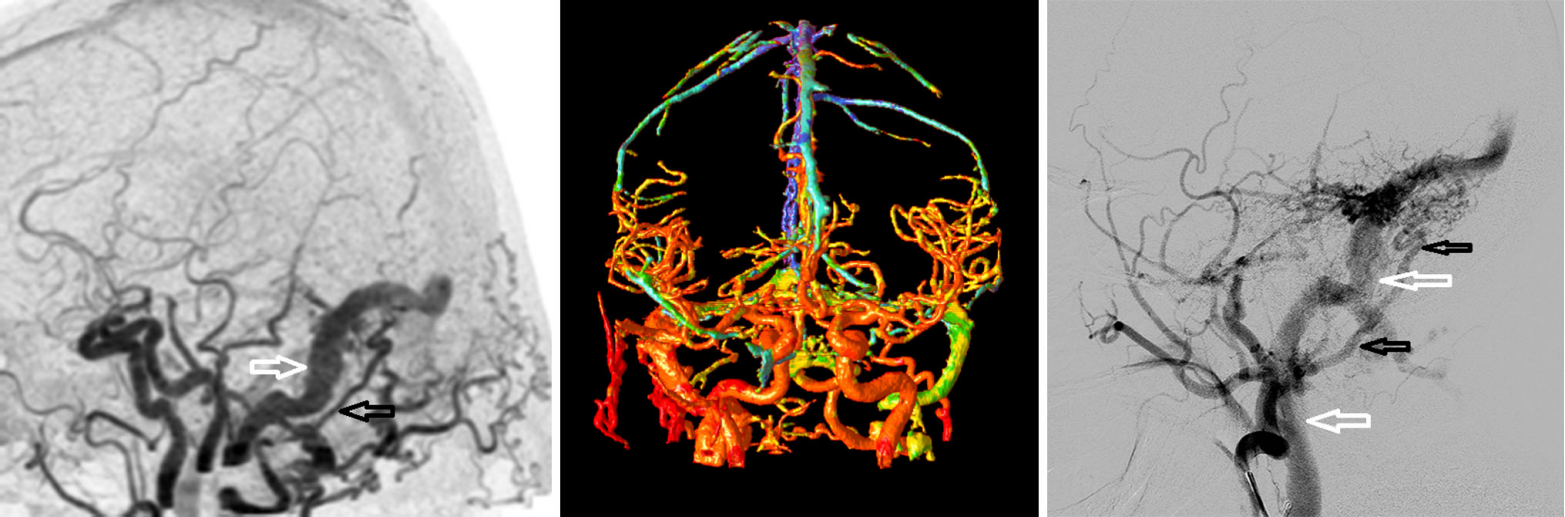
Dural arteriovenous fistula (dAVF) located in the right sigmoid sinus as identified by 4D-CTA and DSA. *Left* 4D-CTA lateral subtracted MIP demonstrating abnormal early contrast filling of the sigmoid sinus (*white arrow*) consistent with dAVF. Hypertrophic occipital artery identified as arterial feeder (*black arrow*). Anterograde venous drainage in the jugular vein. *Middle* Color-coded processing of 4D-CTA. Early contrast enhancement (*arterial flow*) is coded as *red-orange*, delayed contrast enhancement is coded as *yellow-green*. Notice the *red-colored*, hypertrophic occipital artery on the right side serving as arterial feeders of the dAVF. *Right* DSA, selective contrast injection of the external carotid artery showing a hypertrophic tortuous occipital artery (*black arrows*). Venous drainage of the sigmoid sinus into the jugular vein (*white arrows*) (Color figure online)
